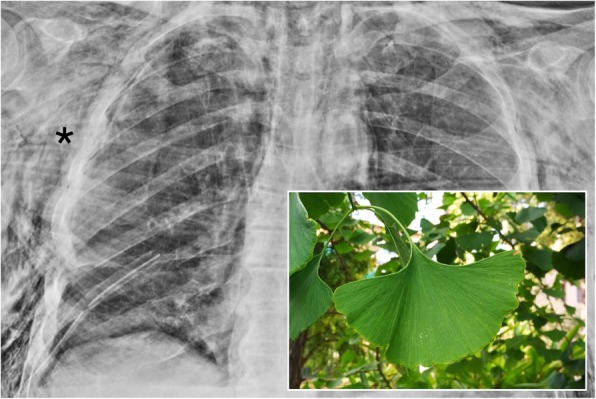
Ginkgo leaf sign. A chest radiograph of a patient with diffuse subcutaneous emphysema. The post-surgical radiograph clearly shows a large amount of air located in the subcutaneous tissues, penetrating into the pectoral muscle fibers (black asterisk). This striated appearance resembles a ginkgo leaf
